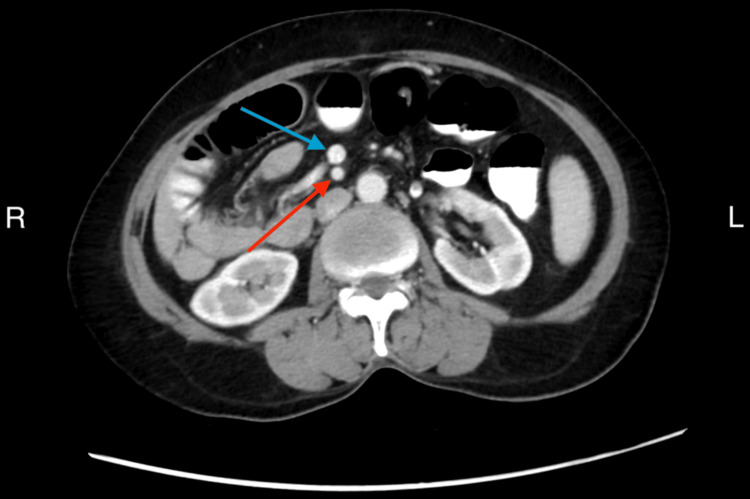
Axial portovenous phase CECT image showing the superior mesenteric artery (red arrow) and superior mesenteric vein (blue arrow) with abnormal anterior position of the vein, in keeping with intestinal malrotation CECT: contrast-enhanced CT
